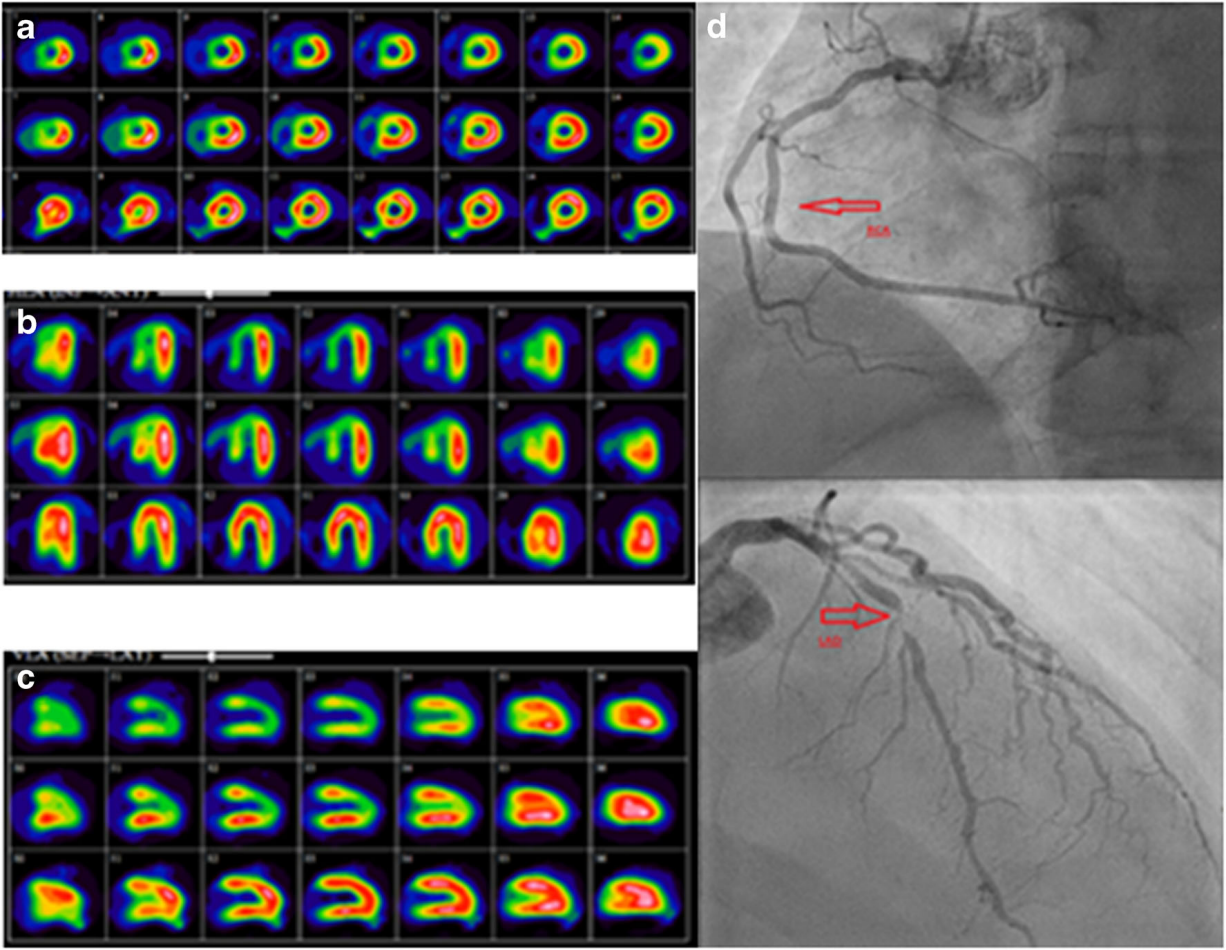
^99m^Tc-tetrofosmin cardiac SPECT/CT for attenuation correction. A patient presenting with chest pain and with family history of CAD was referred for a myocardial perfusion SPECT/CT using a single-tracer one-day stress–rest protocol with ^99m^Tc-tetrofosmin. CT AC was performed for the stress study. MPI-SPECT short (a), horizontal (b), and vertical (c) long axis show moderate reduction in tracer uptake in the anteroseptal wall sparing the anterobasal segment, and in the inferior wall and the apex on the non-corrected images at stress (first row), normalizing at rest (third row), suggests the presence of moderate ischemia in the territories of the left anterior descending (LAD) and right coronary (RCA) arteries. AC stress images (second row) confirm the presence of decreased uptake in the territory of the LAD only (the anteroseptal wall and apex), while correcting the attenuation artefact caused by the diaphragm in the inferior wall. The findings are consistent with a large area of moderate ischemia in the territory supplied by the LAD. Coronary angiography (c) demonstrates normal RCA (top, arrow) and a critical lesion in the proximal LAD (bottom, arrow)
